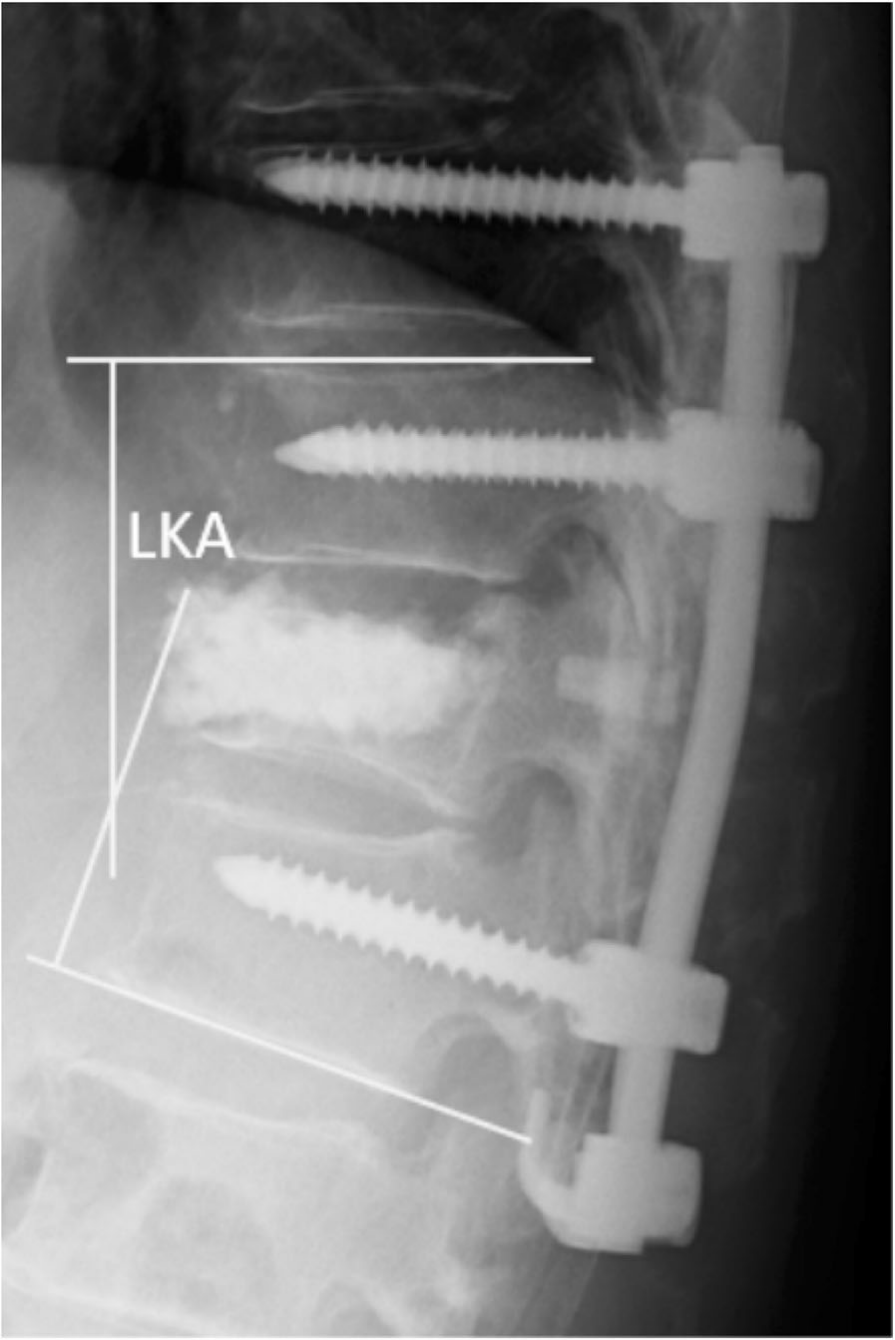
Using lateral radiographs, local kyphosis angle (LKA) was measured between the upper endplate of the vertebra one above the affected level and the lower endplate of the vertebra one below the affected level using the Cobb method
